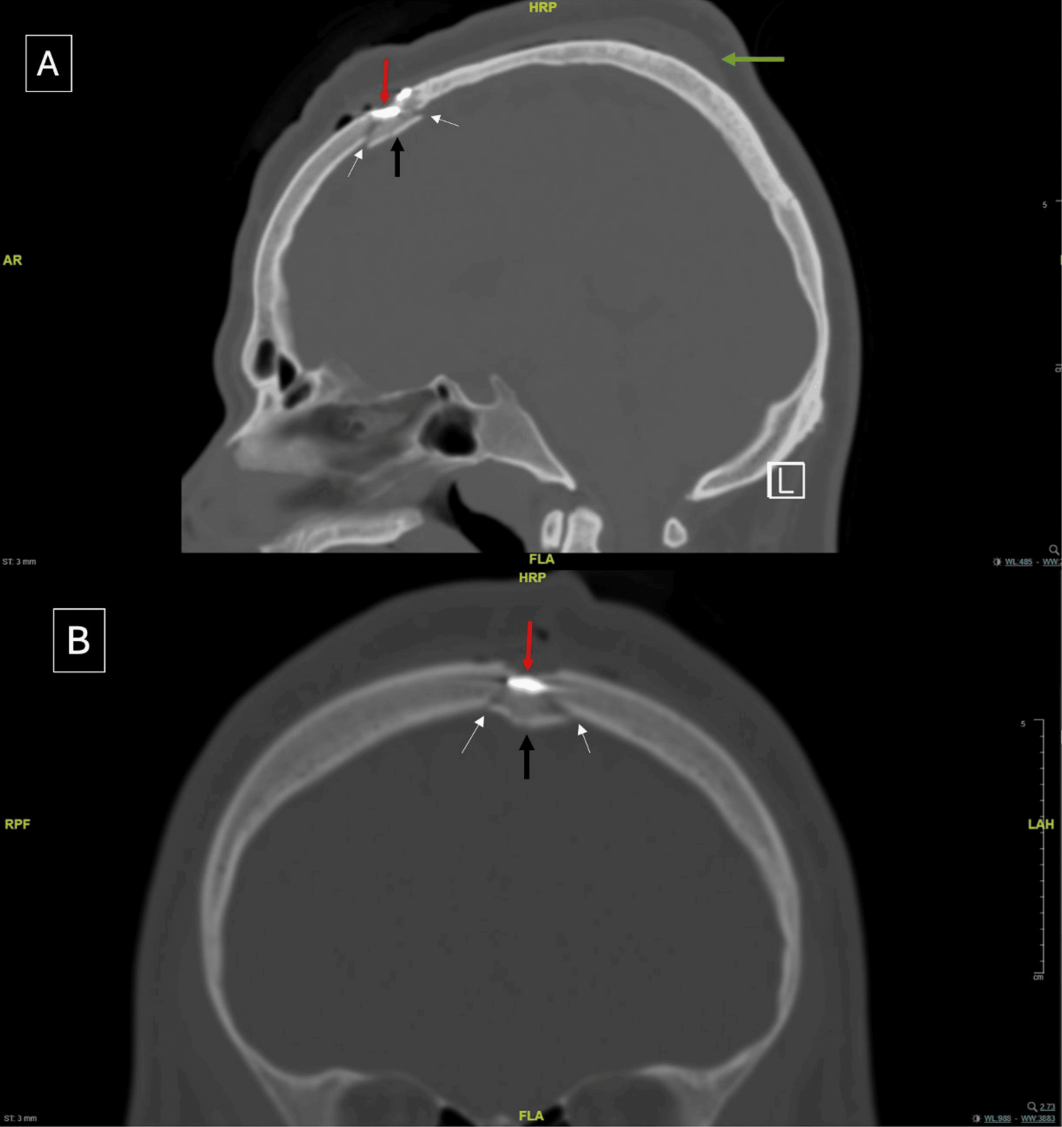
Postoperative non-contrast head CT demonstrating a subgaleal hematoma (green arrow), bullet fragments (red arrow), the bony island (black arrow), and fracture lines (white arrows). (A) Sagittal view. (B) Coronal view CT: computed tomography
